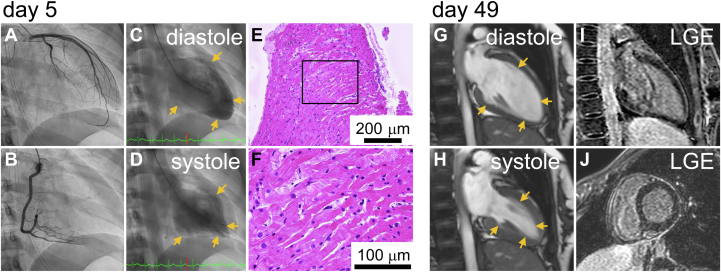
Cardiac Catheterization Performed on Day 5, and Cardiac Magnetic Resonance on Day 49 (A and B) Coronary angiography reveals no abnormalities. (C and D) Left ventriculography clearly shows wall motion typical of reverse takotsubo cardiomyopathy. (E and F) An endomyocardial biopsy shows no edema, inflammation, or fibrosis. Magnetic resonance imaging shows normal left ventricular wall motion (G and H) and no LGE (I and J). Yellow arrows indicate the endomyocardial surface. LGE = late gadolinium enhancement.
